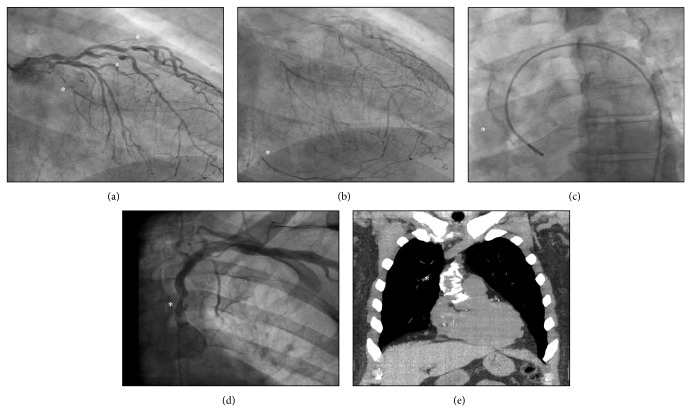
Cardiac catheterization shows multivessel coronary artery disease with occluded left circumflex artery as well as high-grade lesions of the left anterior descending artery and diagonal arteries (a). In addition, delayed imaging shows occluded right coronary artery filling via left-to-right collaterals (b). Cardiac catheterization (c) shows a heavily calcified ascending aorta as well as left subclavian artery stenosis (d). Noncontrast computed tomography of the chest again shows a heavily calcified ascending aorta (e). All lesions are represented with “^∗^”.
